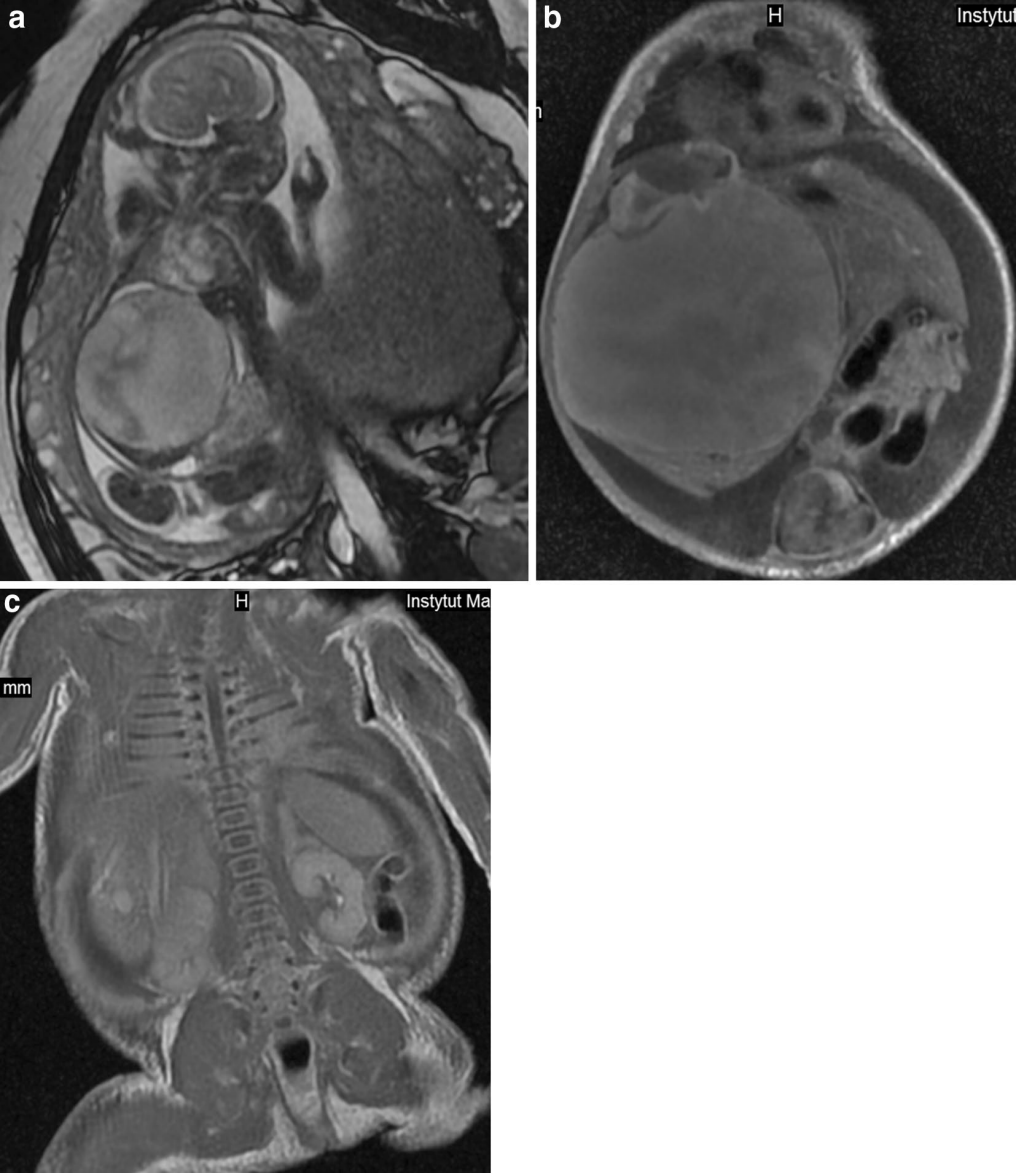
Case no. 11. a Fetal MRI. Huge abdominal and pelvic cyst. b Postnatal MRI. The dominant cyst and a small enhanced nodule in the *left* lobe of the liver. c Postnatal MRI. Enhanced nodules in the *right* lobe of the liver behind the main mass, in the chest wall on the *right side* and in the diaphragm on the *left*
